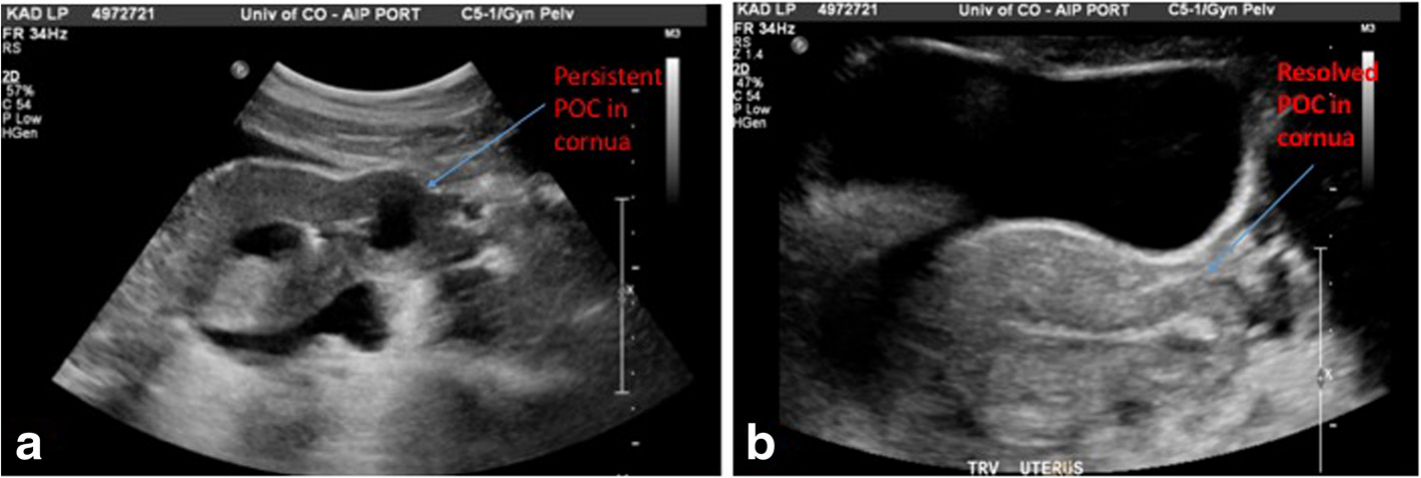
Intraoperative transabdominal ultrasound of Case 2. a Interstitial ectopic pregnancy with a gestational sac in the left cornu and generalized reactive muscular echogenicity surrounding the sac. b) After successful hysteroscopic removal, the decidualized reaction in the left cornu resolves. *POC* products of conception
